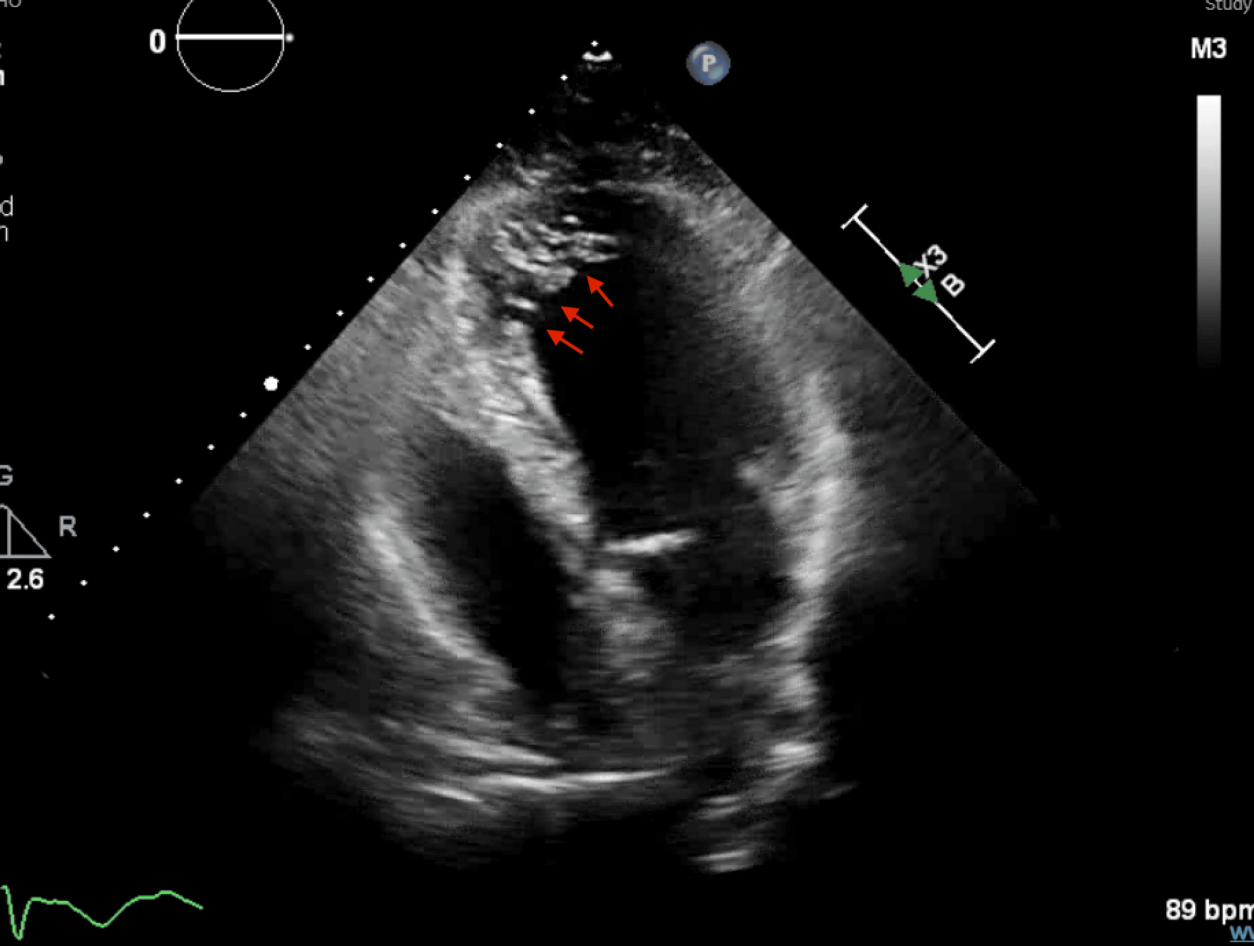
Echocardiographic image at end-systole demonstrating prominent trabeculations with a noncompacted-to-compacted myocardial ratio >2:1 The red arrows indicate some of the excessive trabeculations within the left ventricle. The noncompacted-to-compacted ratio was measured in end-systole using the Jenni criteria, confirming the diagnosis of left ventricular noncompaction.
